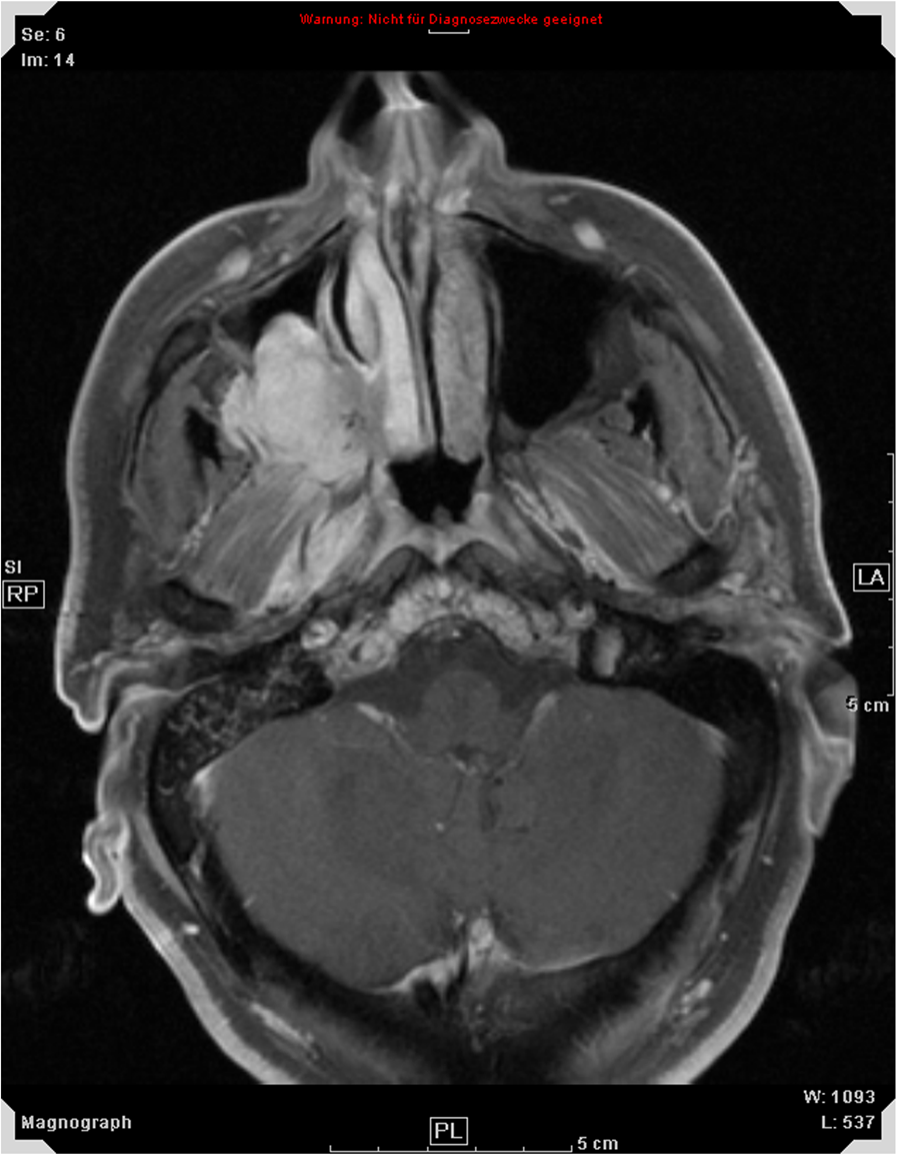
51 year old patient with adenoid cystic carcinoma extending from the right maxillary sinus into the right orbit and cavernous sinus, contrast-enhanced, T1-weighted MRI for treatment planning.
